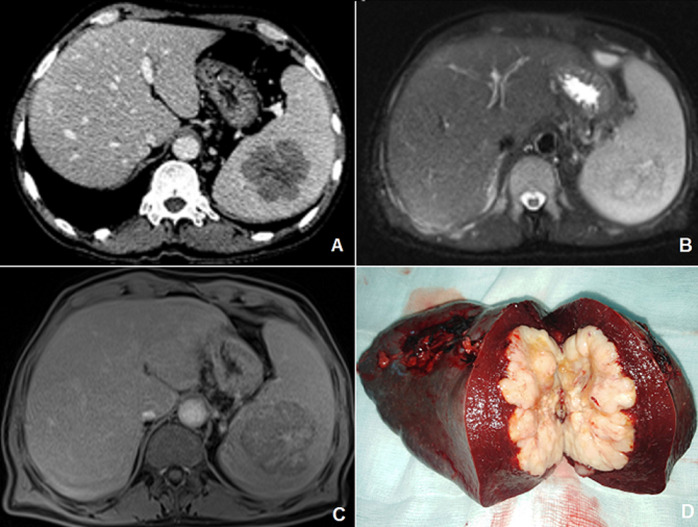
A) CT scan injected during portal phase: hypodense mass slightly enhanced at the periphery, measuring 57x60x65mm and deforming the splenic hilum, without splenomegaly nor lymph node; B, C) magnetic resonance imaging found a polylobed lesion in T1-T2 isosignal, diffusion hypersignal, crossed by fibrous spans and delimited by a thin wall in T2 hypointense taking the contrast; D) intra-splenic tumor mass with a whitish macroscopic appearance, with polycyclic contours and crossed by fibrous partitions
